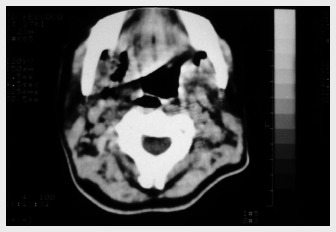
Computed tomography scan of cervical region showing bilateral lateropharyngeal and retropharyngeal collections.
